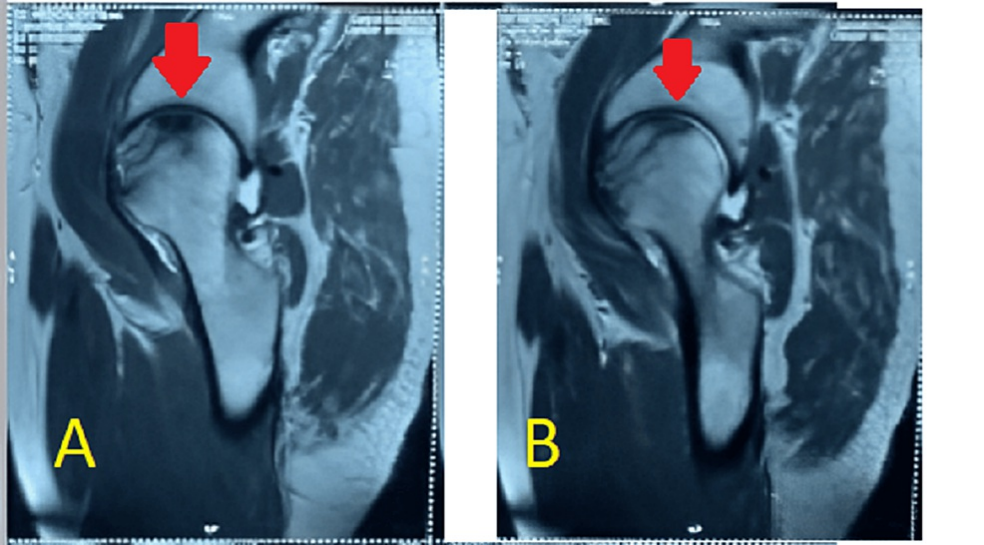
Sagittal section (A) and (B) of MRI, both hips showing destruction on left side The sagittal slices of images A and B show avascular necrosis, or the loss of vascularity, on the left side.
